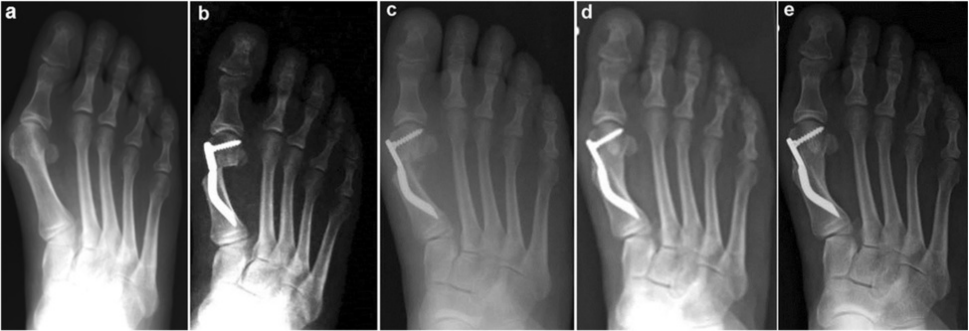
Case 4. A 51-year-old woman with moderate HV; antero-posterior radiographic images at: a Preoperative period. b 1-month follow-up. c 3-month follow-up. d 6-month follow-up. e 48-month follow-up showing the maintained correction of the deformity
